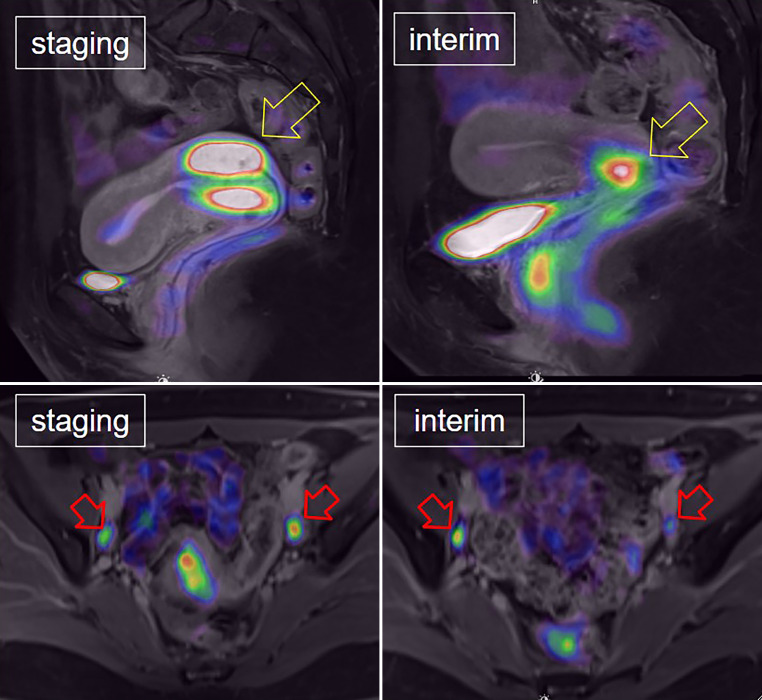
In a patient with cervical cancer, an incomplete metabolic response was observed. This was characterized by the persistence of FDG uptake in both the primary cervical cancer lesion (yellow arrow) and the bilateral pelvic lymph node metastases (red arrows)
